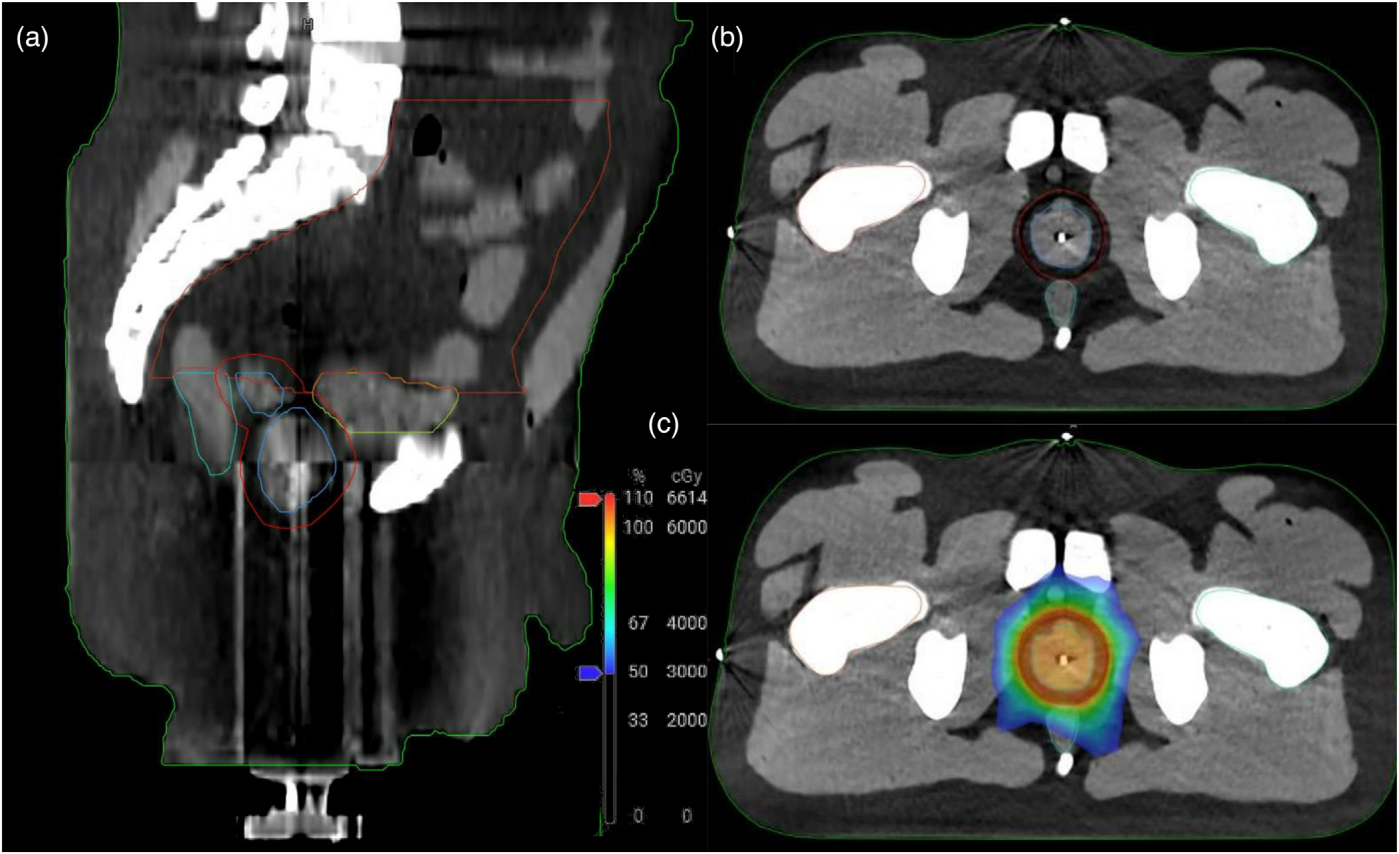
(a, b) Orthogonal views of contoured structures used to generate reference and adapted plans. Imaging was acquired using Varian HyperSight CBCT. (c) Axial view of the dose distribution for the 60 Gy in 20 fraction plan generated in Ethos. The displayed contours are: CTV (blue), PTV (red), bowel bag (orange), bladder (yellow), rectum (teal), external body (green).
